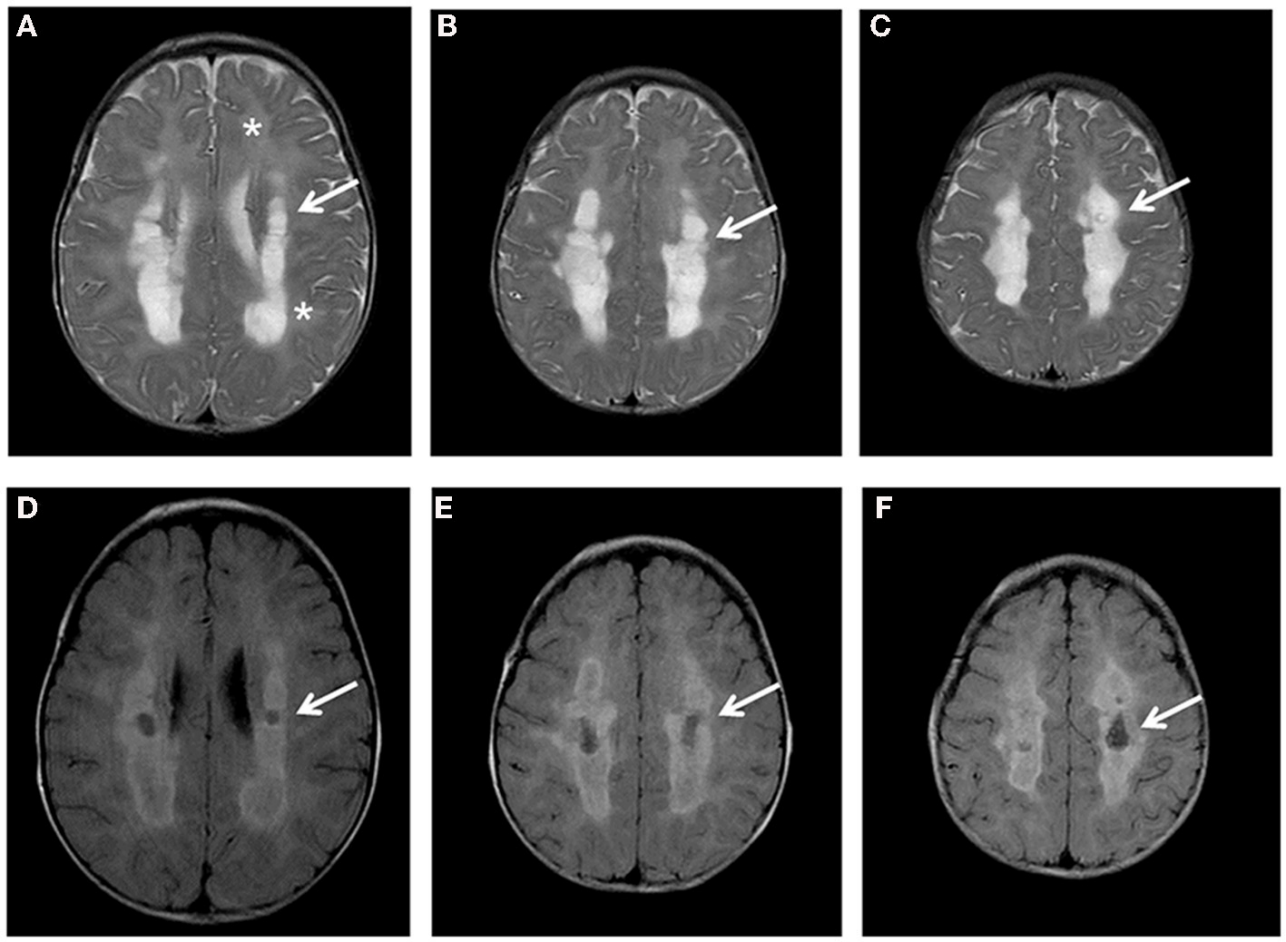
Brain MRI. Axial T2 (A-C) and FLAIR images (D-F) show diffuse hyper intensity of the white matter (A: asterisks) more prominent in the posterior periventricular and deep regions (A-C: arrows) with evidence of partial cystic degeneration and cavitations in FLAIR sections (D-F: arrows).
